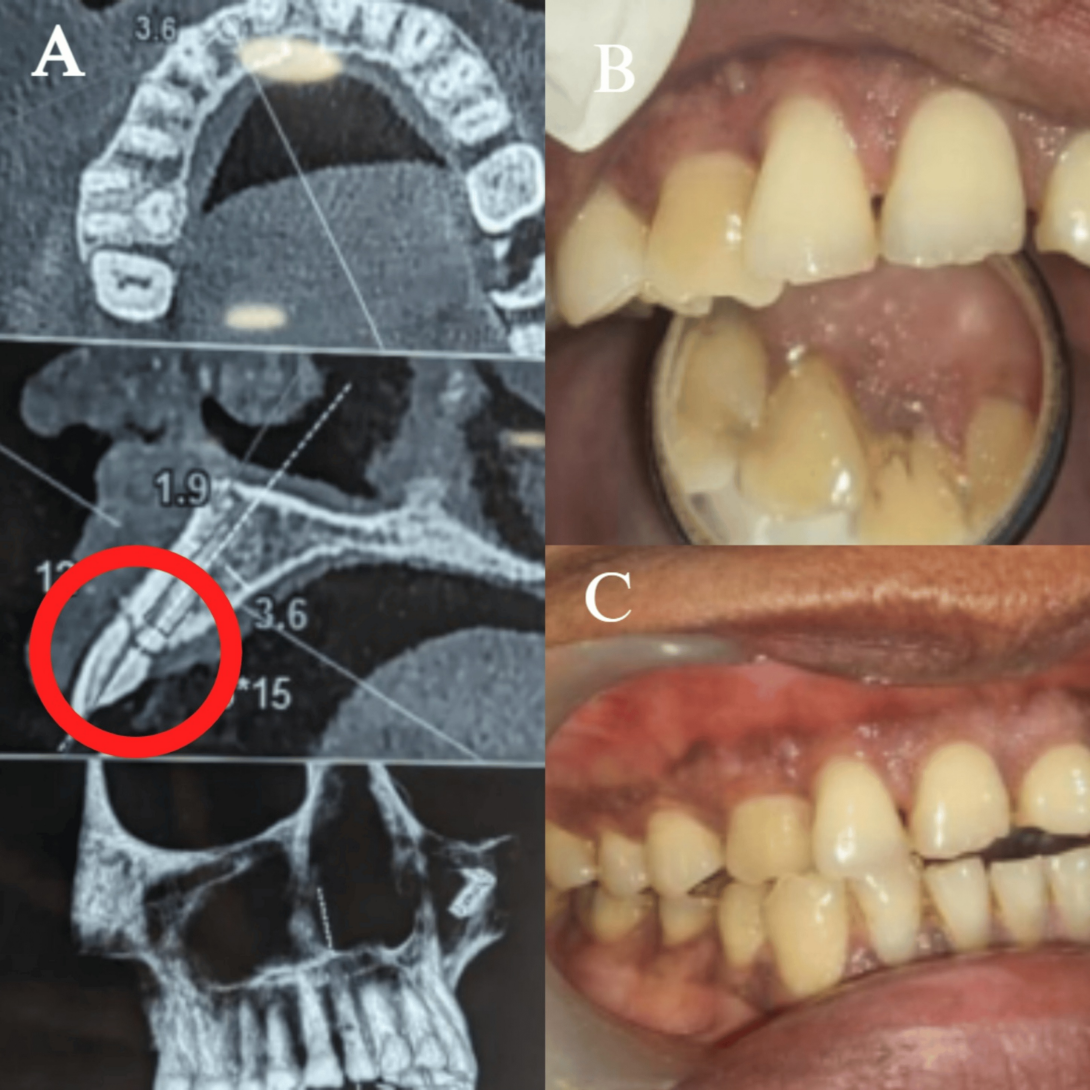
(A) CBCT scan showcasing Ellis Class VIII fracture; (B, C) intraoral images showcasing discolored tooth (#12) (A) CBCT scanned image in relation to #12, which shows a fracture line at the cervical region; (B, C) clinical image of the fractured, discolored tooth CBCT: cone-beam computed tomography
